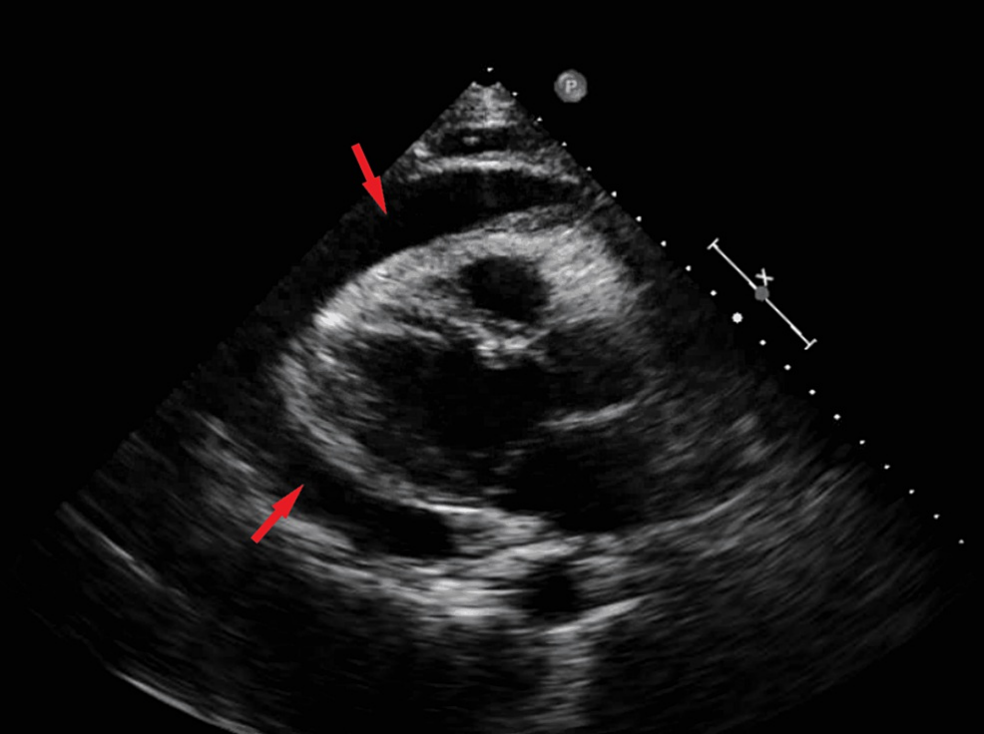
Transthoracic echocardiogram showed large pericardial effusion (red arrows).
